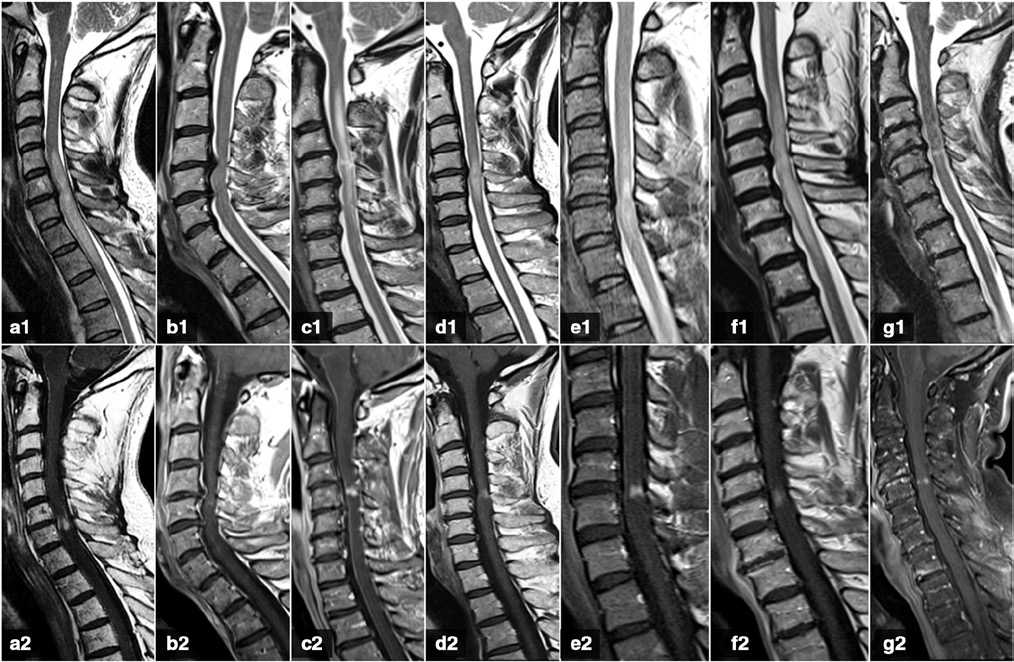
First MRI scans from our case series, sagittal plane. Sagittal T2-WI (A1-G1) and post-contrast T1-WI (A2-G2) show intramedullary spindle-shaped T2-signal hyperintensities in cases 1, 3, 4, 5 and 6 (A1, C1-F1) and focal-shaped in cases 2 and 7 (B1, G1). Also, gadolinium enhancement is seen as a transverse band in cases 1, 3, 4 and 7 (A2, C2, D2, G2); and a focal enhancement in cases 2, 5 and 6 (B2, E2, F2).
